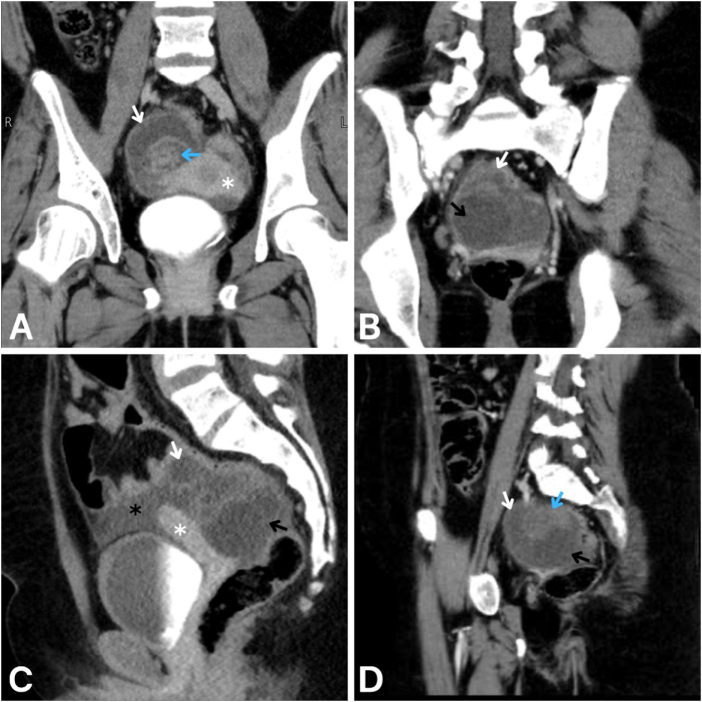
Contrast-enhanced abdominal CT showing the adnexal lesion in coronal (A and B), sagittal (C), and oblique (D) planes. (A) The upper segment of the hydrosalpinx (white arrow) is seen above the whirlpool sign of the twisted pedicle (blue arrow). The uterus is also visible (white asterisk). (B) Both the lower segment of the hydrosalpinx (black arrow) and the upper segment (white arrow) are shown. (C) Sagittal plane showing the spatial relationship between the lower segment of the hydrosalpinx (black arrow), the upper segment (white arrow), and the uterus (white asterisk). A small amount of free fluid is present (black asterisk). (D) Oblique view showing continuity between the two hydrosalpinx segments (white and black arrows), with the whirlpool sign centrally located (blue arrow).
